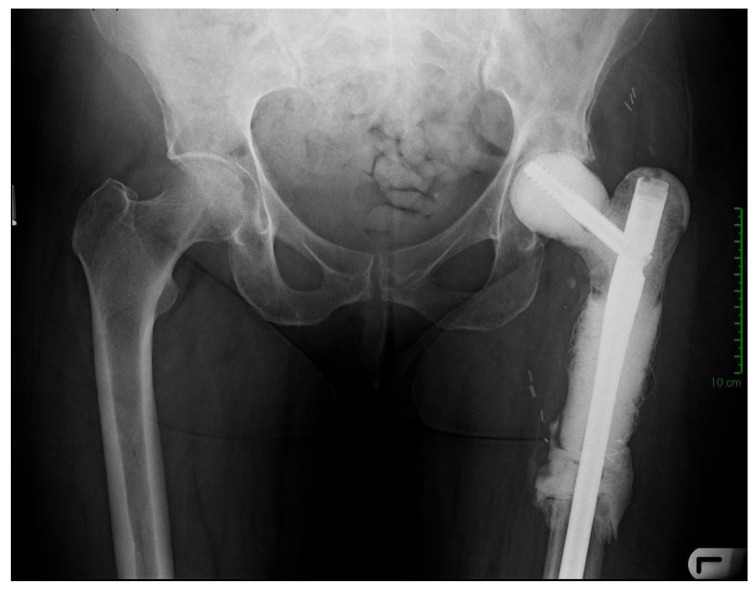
Postoperative x-ray following 1st stage revision.
